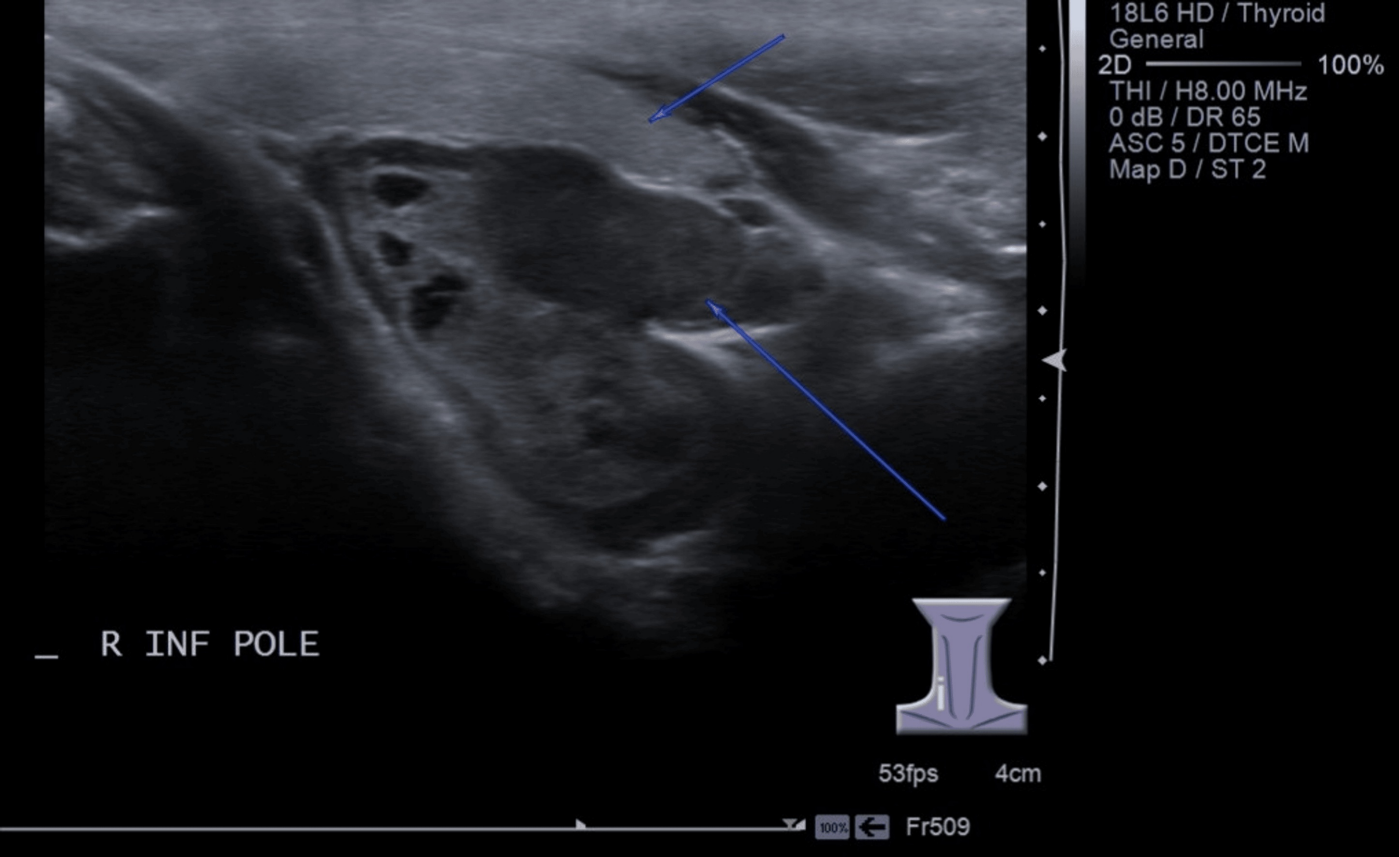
Ultrasound scan (longitudinal) demonstrating well-defined mixed echogenicity solid cystic lesion located posterior to the inferior pole of the right thyroid gland. The short blue arrow shows a homogenous right thyroid lobe, and the long blue arrow shows a heterogeneous lobulated parathyroid nodule.
